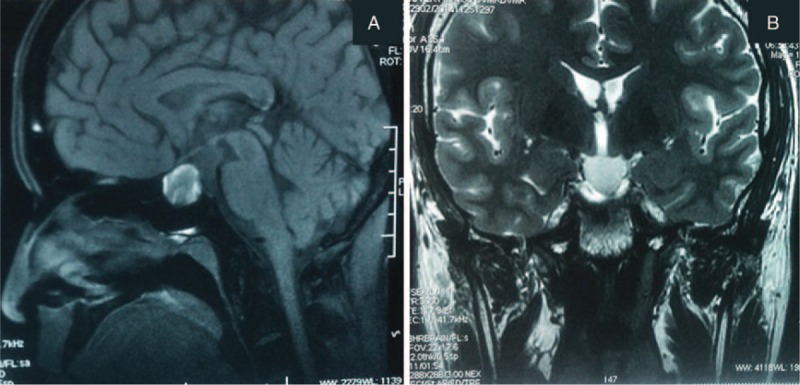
MRI evaluation of the sellar region before surgery. The mass measured 13 mm × 13 mm × 18 mm. The lesion's interior was cystic, which showed as isointense gray matter mixed with hyperintensity on T1-weighted image (A) and showed iso-high T2 signal (B).
